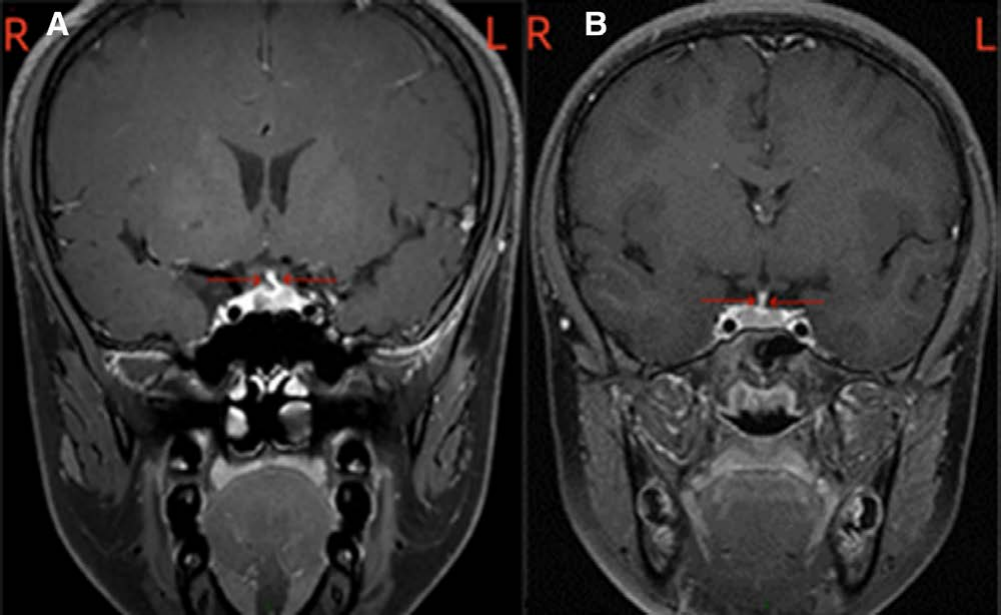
Pituitary stalk tilting: MRI direct dynamic enhancement scan of the pituitary gland: (A) the pituitary gland has a diameter of approximately 7.3 mm, and the pituitary stalk deviates to the left. (B) The median height diameter of the pituitary gland was approximately 6 mm, and the pituitary stalk was slightly to the right. MRI = magnetic resonance imaging.
